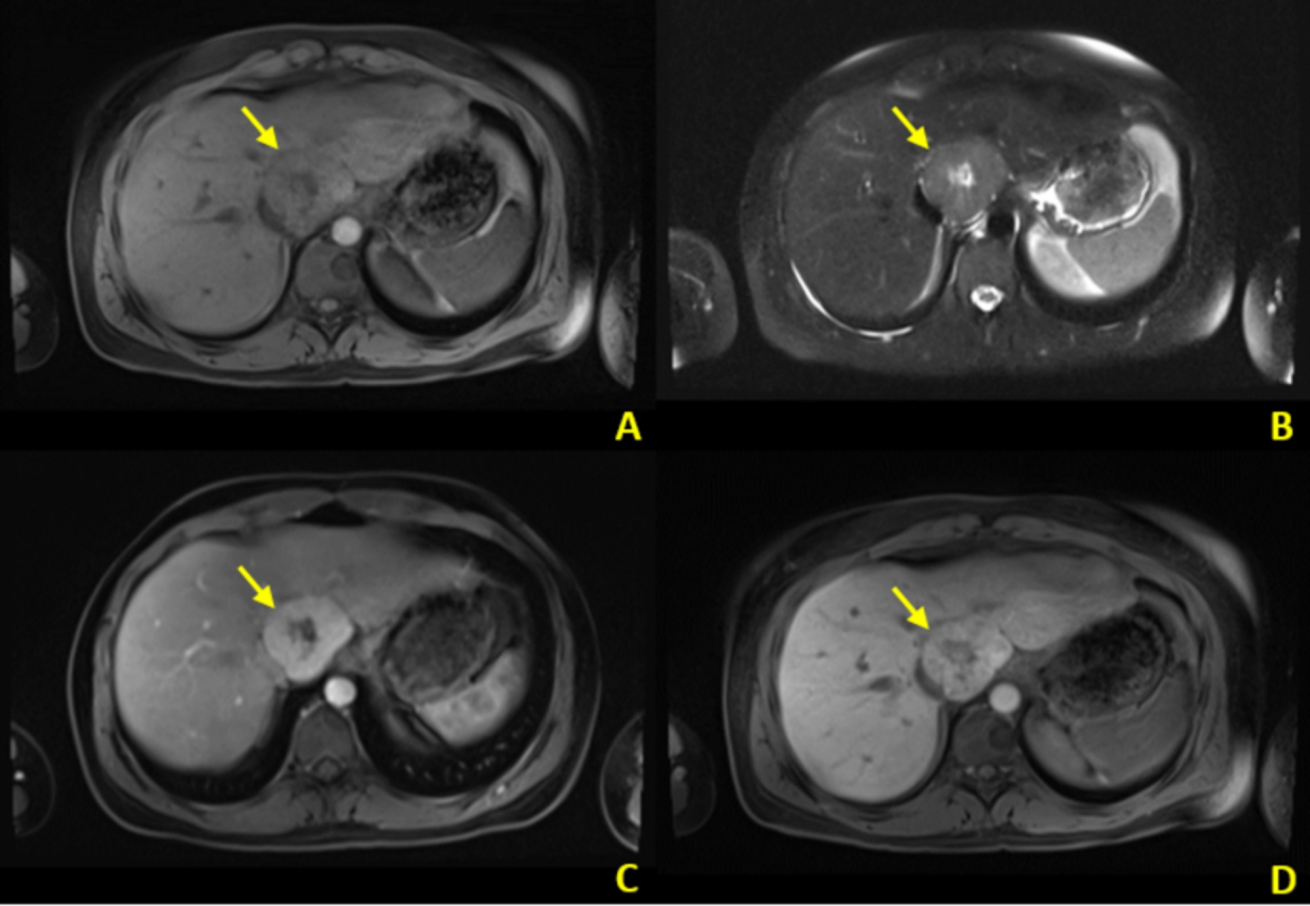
Contrast-enhanced abdominal MRI MRI: magnetic resonance imaging Contrast-enhanced (hepatobiliary agent) MRI of the same patient. A. T1 axial MR image demonstrates T1 isointense signal peripherally with a central focus of T1 hypointensity. B. T2 axial MRI demonstrates central T2 hyperintensity with relatively decreased signal in the periphery. C. Contrast-enhanced T1 MRI in bolus phase demonstrates peripheral enhancement of the mass. D. Contrast-enhanced T1 MR image in delayed/hepatobiliary phase demonstrates persistent contrast enhancement. Imaging characteristics favor FNH and confirm CT findings
